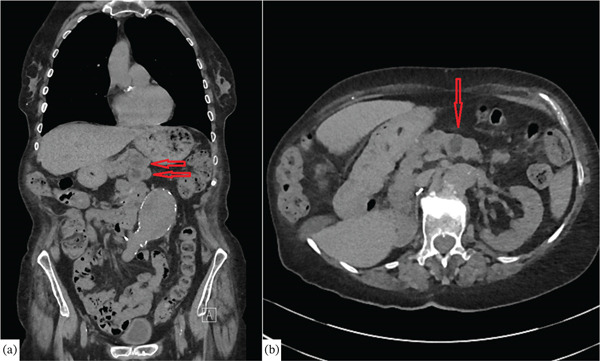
Abdominal CT scan findings consistent with pancreatic metastases (red arrow)—(a) coronal view; (b) axial view.
